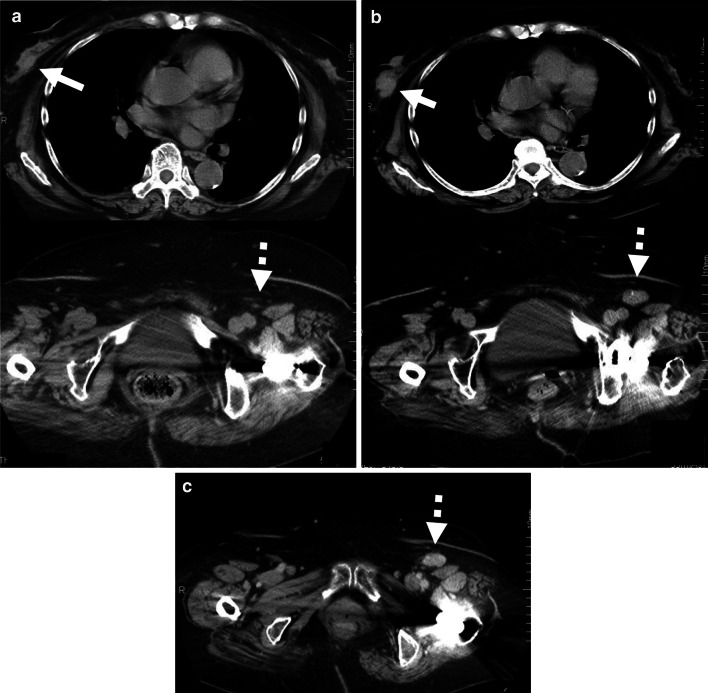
a The chest (above) and pelvic (below) CTs in 28 months before the breast cancer operation: right breast tumor can be barely identified (arrow), whereas left inguinal node swelling cannot be detected (dotted arrow). b The chest (above) and pelvic (below) CTs at the time of breast cancer operation: right breast tumor was getting to be apparent (arrow) in concordance with the left inguinal node swelling, 28 × 17 mm in size (dotted arrow) which had been overlooked. c The pelvic CT in 3 years after the breast cancer operation. As compared with the inguinal swelling shown in b (at the time of breast cancer surgery), the size of the nodes had still been dormant (dotted arrow). During these periods, adjuvant hormone therapy had been done in parallel
